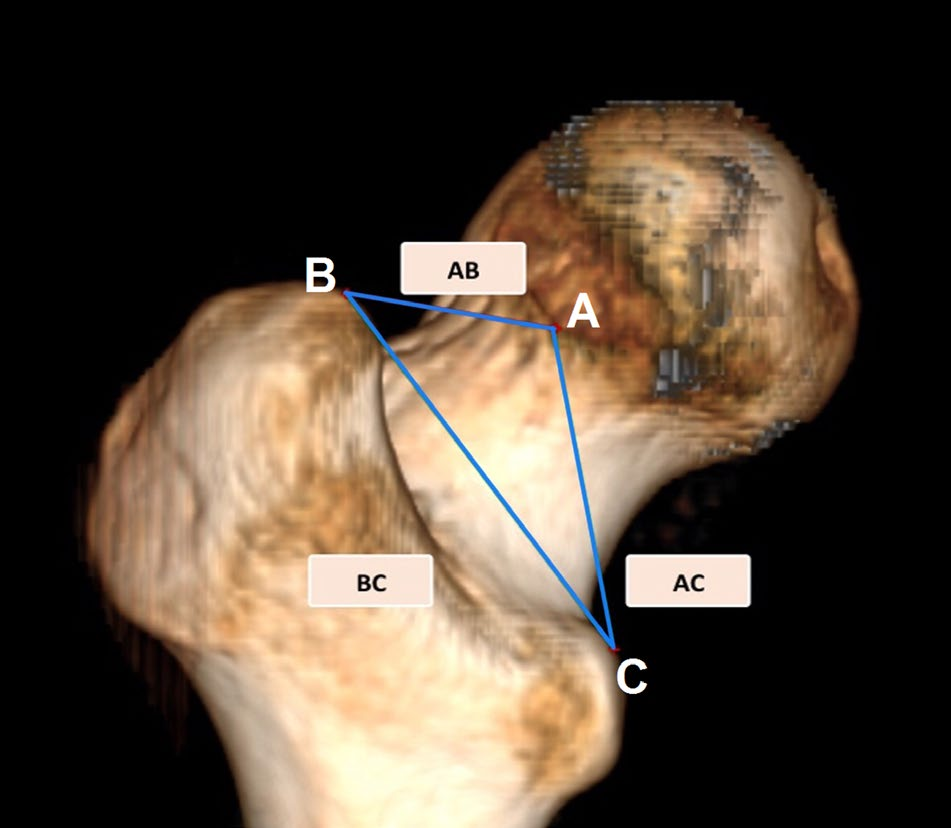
The three linear measurements of Purkait’s triangle labelled on the posterior aspect of 3D volume rendered CT image of left Egyptian femur. The point B is located on the greater trochanter and it is projecting most medially. Point C is the highest point projecting most medially on the lesser trochanter, while point A was located on the articular margin of head dipping most laterally.
